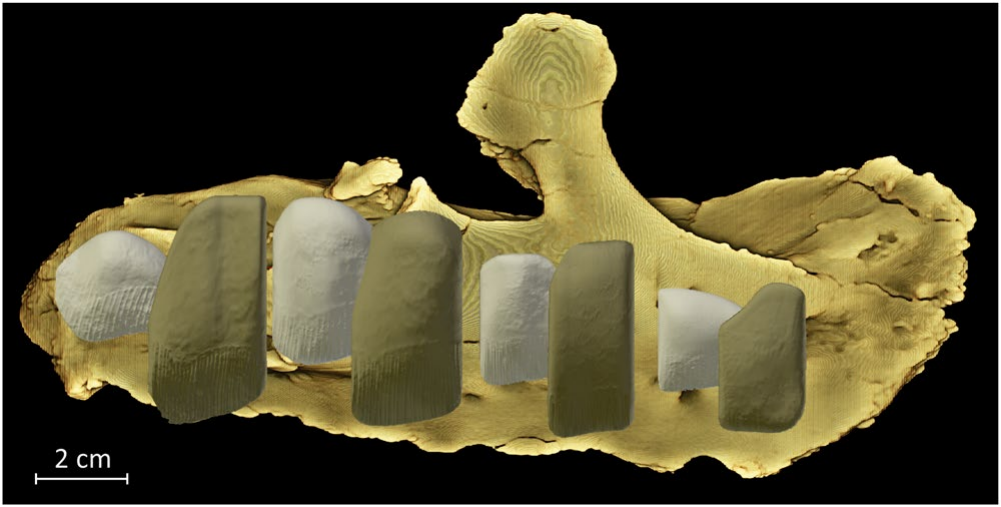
Reconstruction of the maxillary dentition of *Matheronodon provincialis* gen. et sp. nov. in lateral view from CT scans of MMS/VBN-02-102.
